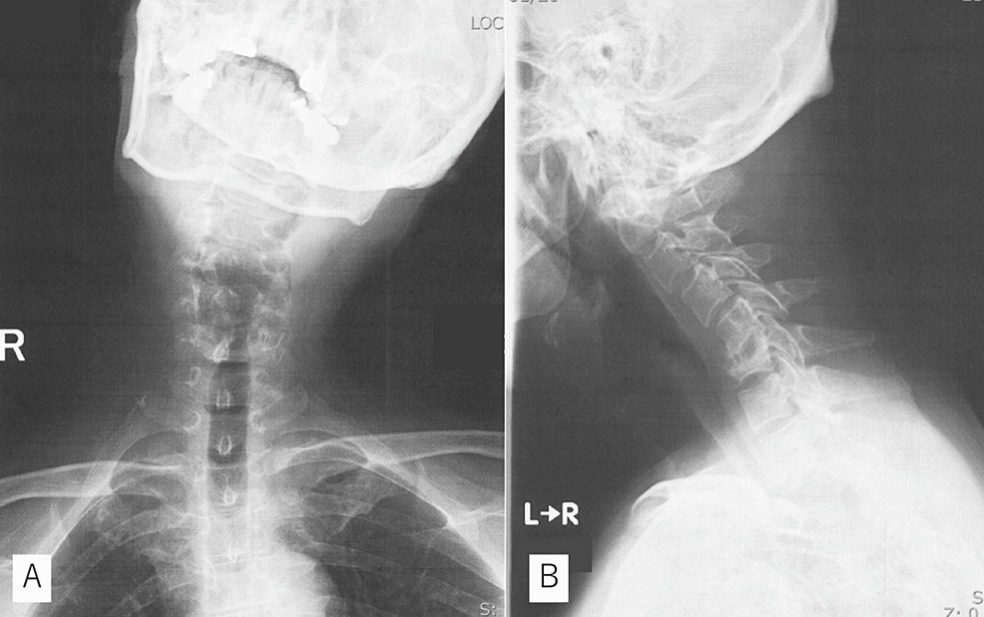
Preoperative X-ray X-ray showing flexion-rotation deformity (A) and wedge deformity at C6 (B).
